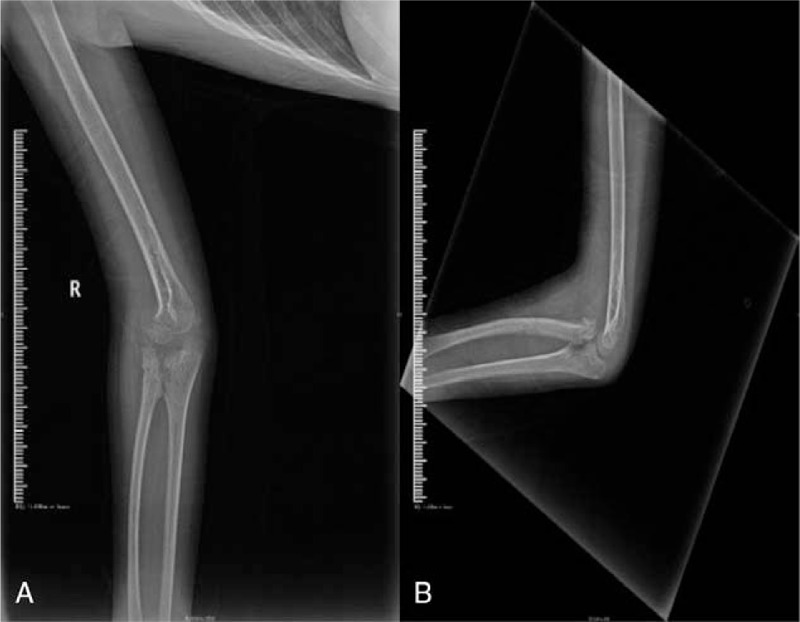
(A) X-ray of the anteroposterior elbow at the 2-mo postoperative follow-up. (B) X-ray of the lateral elbow at the 2-mo postoperative follow-up.
